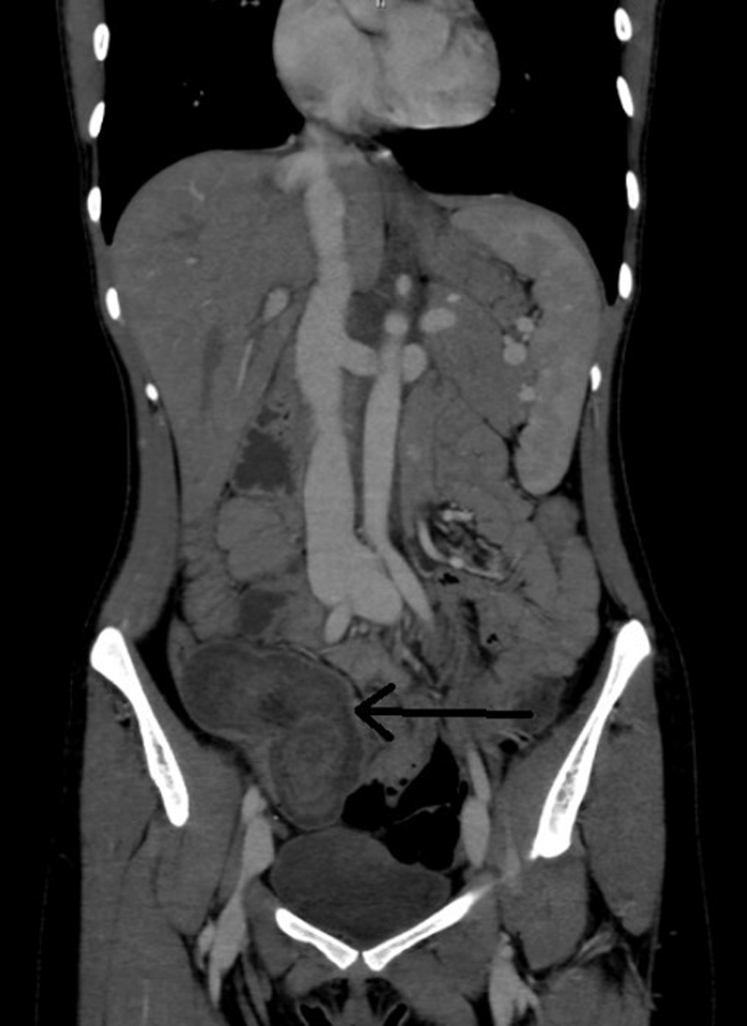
Coronal view of the 3-layered structure in the ileocecal region suggesting intussusception on the patient's computed tomography (ring structure at the tip of the black arrow).
